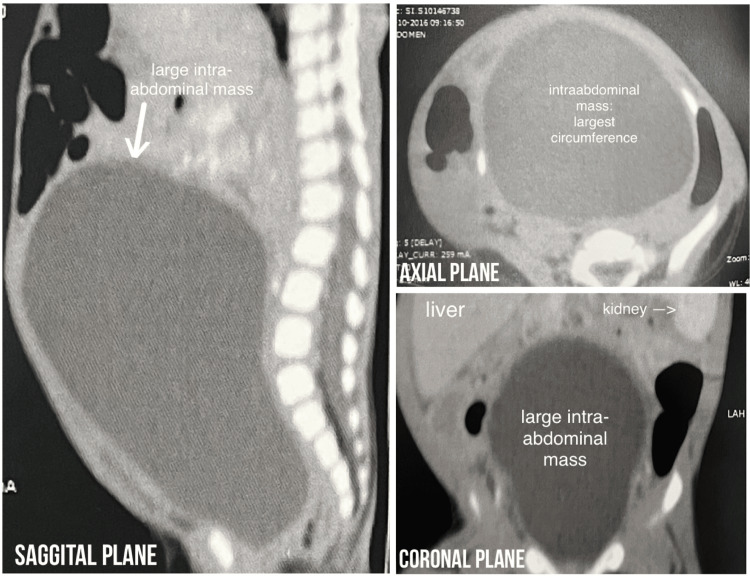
Computed tomography (CT) scan of the patient done in the fourth month of life. Computed tomography (CT) scan of the abdomen and pelvis revealed a large cystic lesion measuring 10 x 6 x 7 cm in the craniocaudal, transverse, and anteroposterior axis, displacing both the urinary bladder and the uterus anteriorly and cranially while also displacing mesenteric vessels and small bowel loops towards its periphery with minimal fluid in the endometrial cavity. This cystic lesion was also causing a mass effect on the distal pelvic ureters, hence causing bilateral hydronephrosis and hydroureters.
